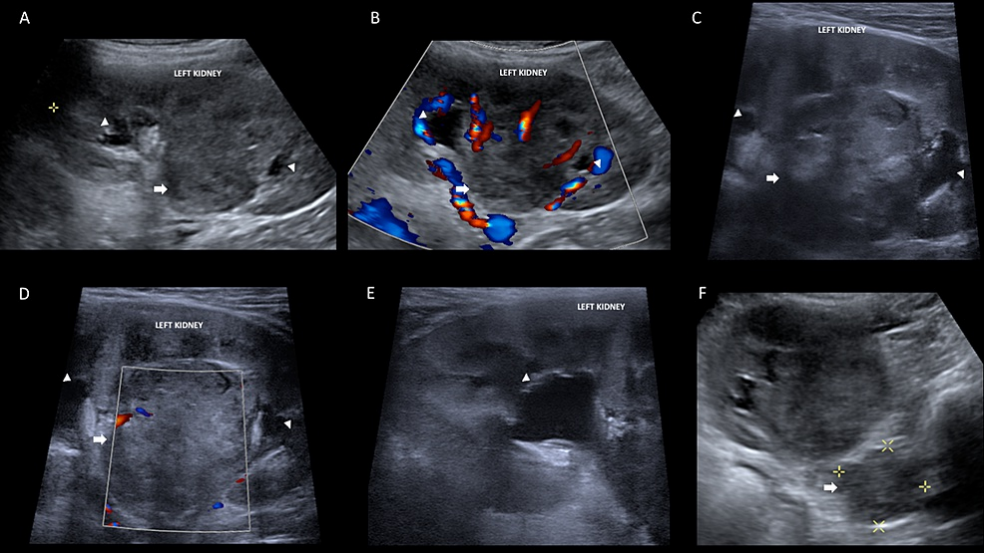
Abdominal ultrasound. Abdomen ultrasound with low-frequency convex transducer (A and F) and high-frequency linear transducer (C and E). Solid renal lesion of central location (arrow in A, B, and C), generating focal caliectasis in both superior and inferior pole (arrowhead in A, B, C, D, and E). It was hypovascular in color Doppler mode (B and D). Additionally, retroperitoneal lymphadenopathies were observed in paraaortic and renal hilum locations (arrow in F).
